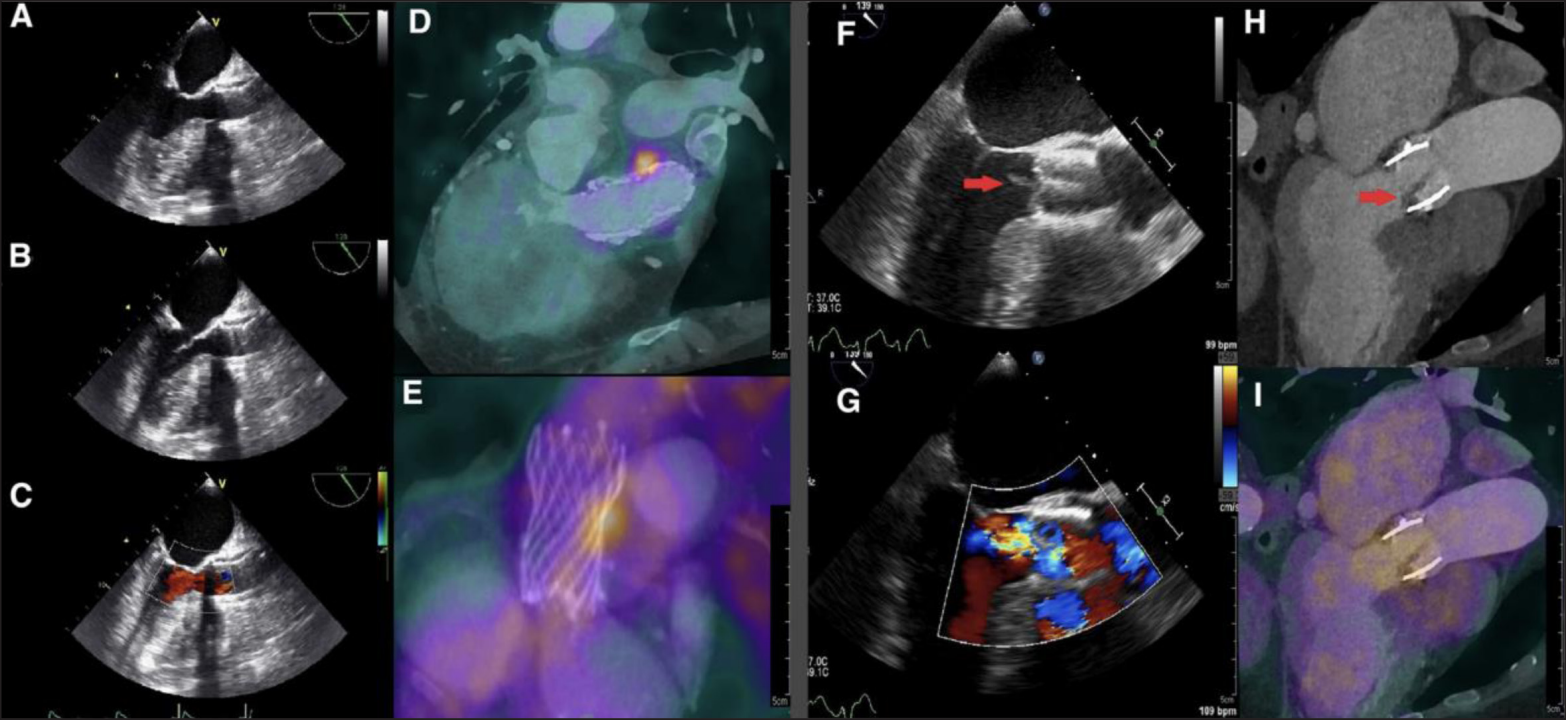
Two cases of one positive PET/CT and one negative PET/CT for TAVI-IE. Case 1 (A to E): A 75-year-old female with suspected Corevalve TAVI-IE underwent a TEE without signs of endocarditis (A to C). PET/CT images (D/E) demonstrated focal FDG uptake alongside the Corevalve as positive sign of TAVI-IE. This case was previously published as a case report. Reprinted with permission.^19^ Case 2 (F to I): An 81-year-old female with suspected Edwards-Sapien TAVI-IE underwent a TEE (F/G) with a vegetation on the aortic valve and mild aortic regurgitation. CTA demonstrated thickening of the aortic valve leaflets (H) as possible signs of vegetation. However, PET/CT images (I) showed no focal 18F-FDG uptake on the leaflets. This was explained by the low inflammatory activity and 2 weeks of intravenous antibiotic therapy prior to the PET/CT scan. Reprinted with permission.^20^ PET/CT: positron emission tomography/computed tomography; TAVI-IE: transcatheter aortic valve implantation-infective endocarditis; FDG: fludeoxyglucose F18; TEE: transesophageal echocardiography; CTA: computed tomography angiography
